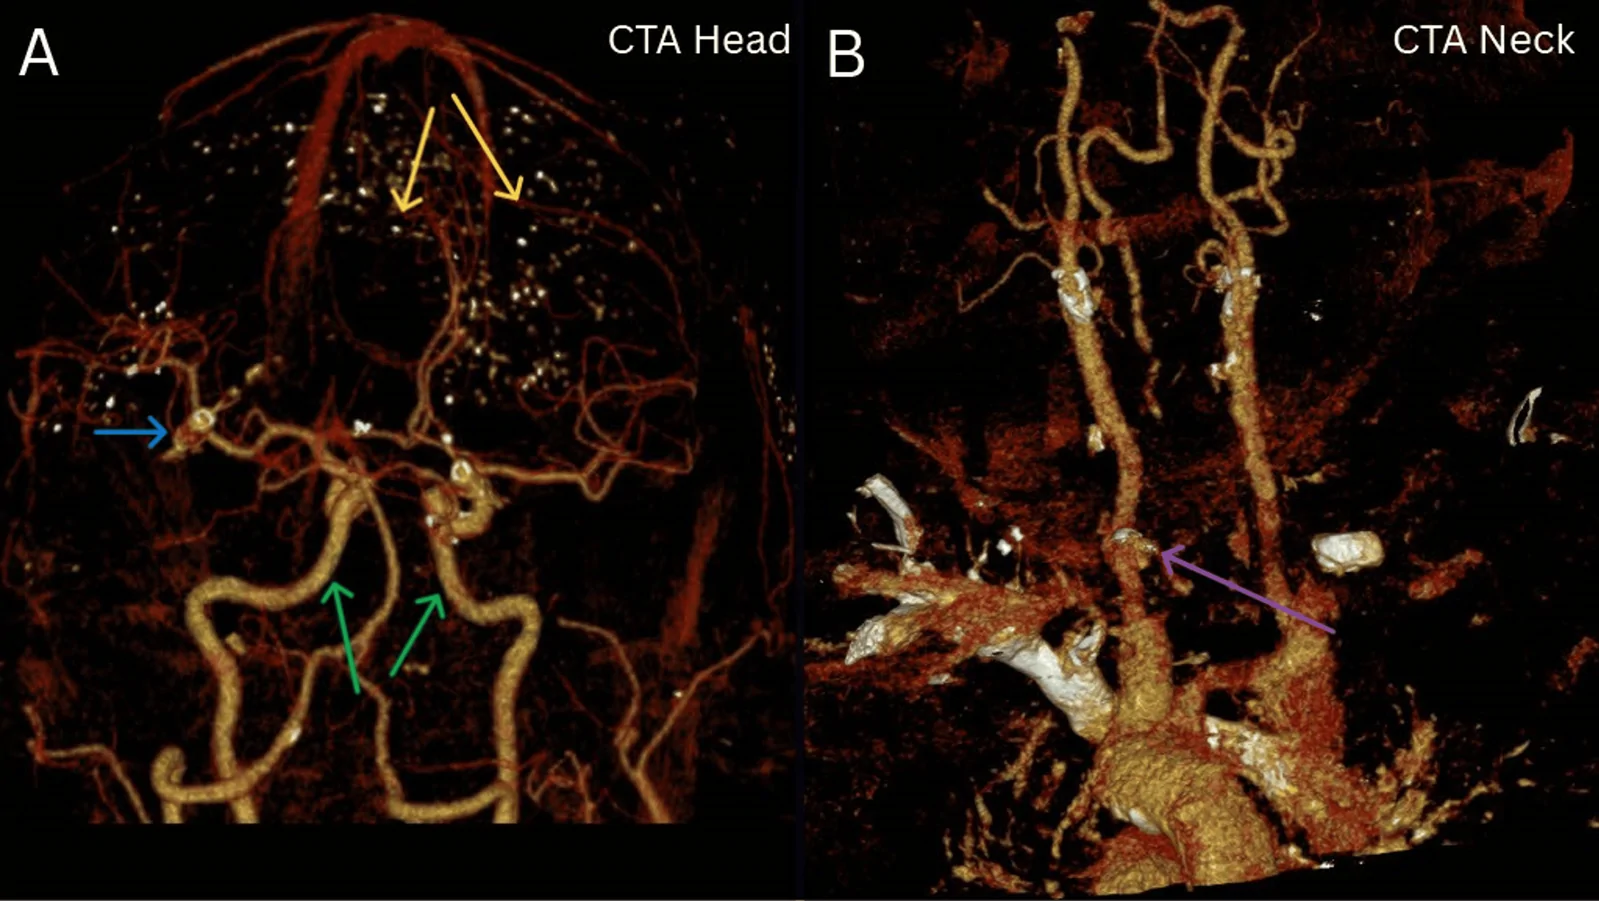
Three-dimensional volume-rendered CTA images of the head (A) and neck (B) demonstrate normal opacification and course of the major arterial vasculature. In A, the intracranial circulation is visualized, including the anterior cerebral arteries (yellow arrows), middle cerebral arteries (blue arrow), and internal carotid arteries (green arrows). In B, the cervical vasculature shows the common carotid arteries (purple arrow) with normal caliber and bifurcation. There is no evidence of significant stenosis, aneurysm, dissection, or vascular malformation CTA: computed tomography angiography
